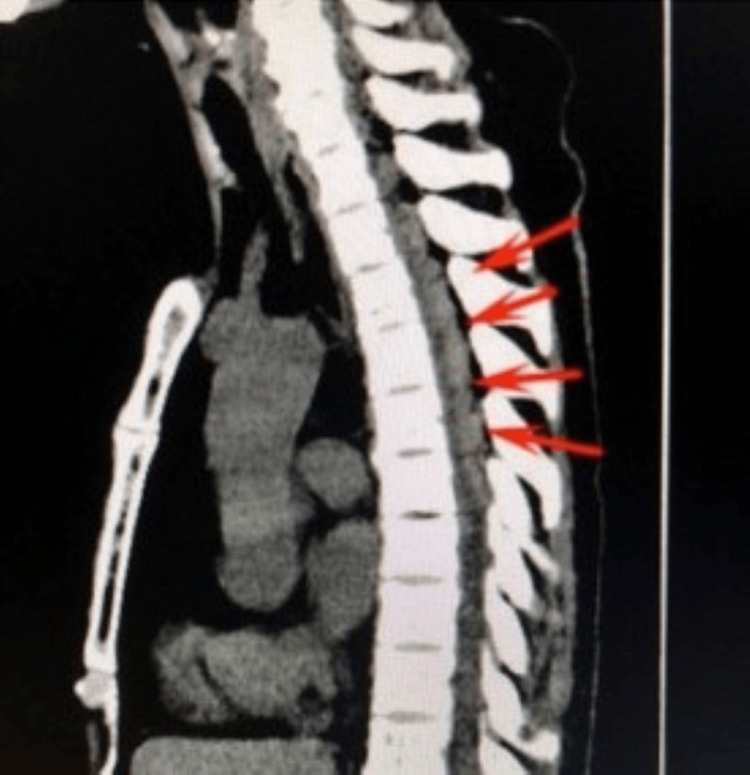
Thoracic epidural hematoma from T3 to T6 with spinal cord compression observed on CT scan performed at 9:15 a.m. on postoperative day 3. Arrows indicate the location of the hematoma.
